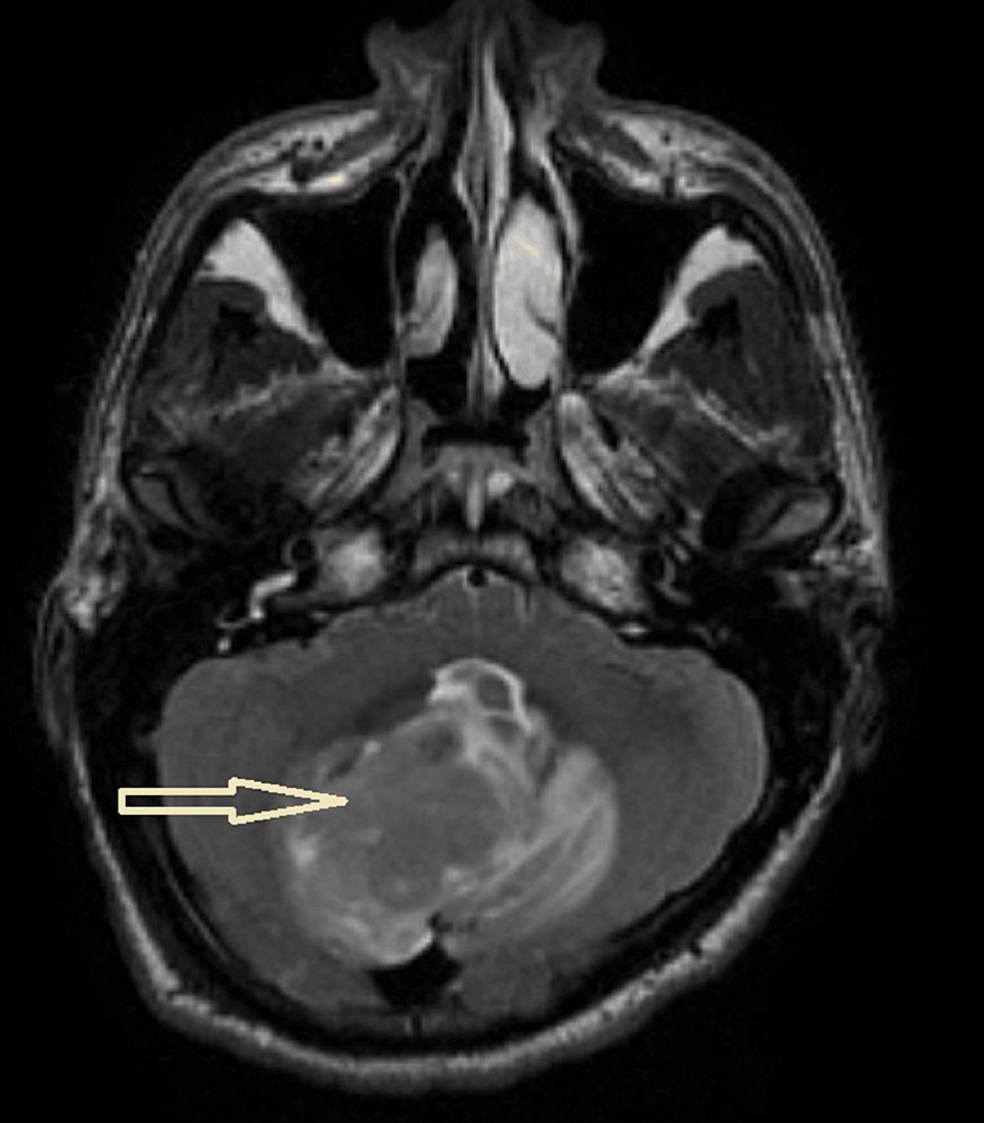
MRI of the brain MRI of the brain demonstrates a large intra-axial/infra-tentorial mass, mainly involving the right cerebellar hemisphere (arrow)
